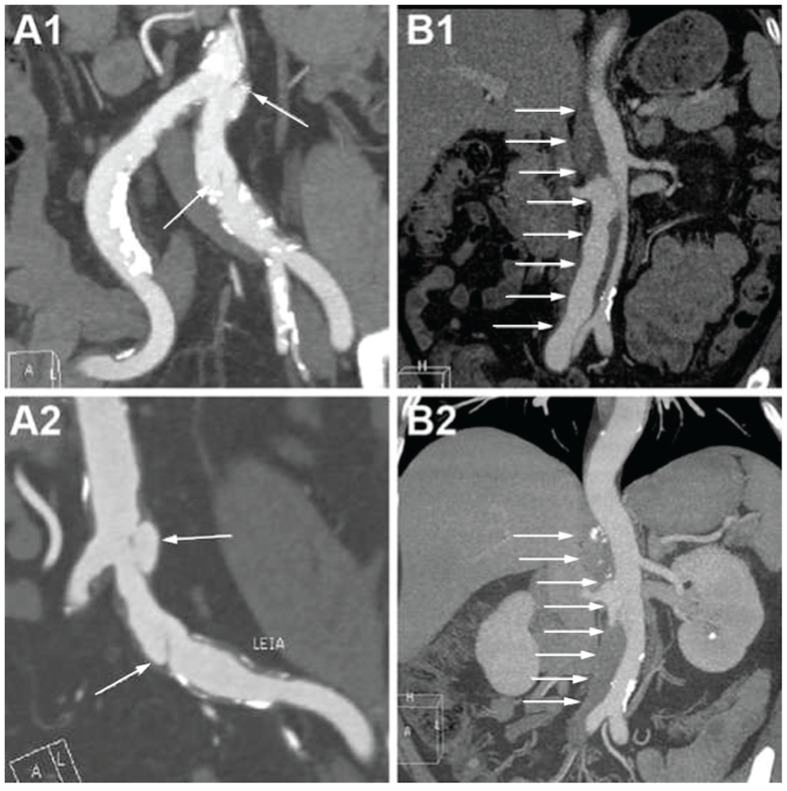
CTA images on admission and at follow-up in focal and extensive SIAAD. (A1) F-SIAAD on admission; (A2) F-SIAAD remained unchanged at 24 months after discharge; (B1) E-SIAAD on admission; (B2) the false lumen of E-SIAAD was almost completely thrombotic at 24 months after discharge. White arrows denote the intimal flap and false lumen. CTA, computed tomography angiography; SIAAD, spontaneous isolated abdominal aortic dissection.
